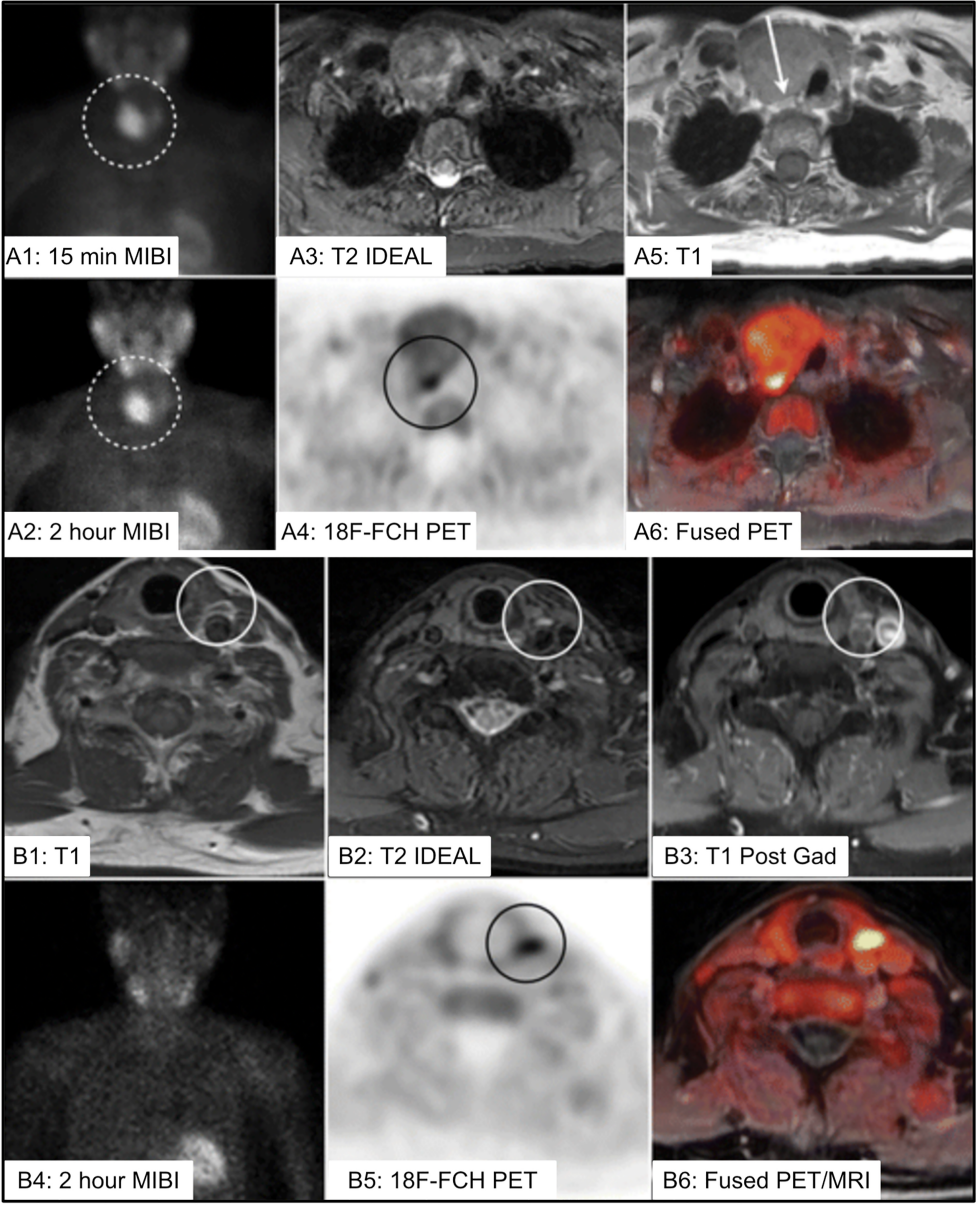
(A) 73 yo F with multinodular goiter and 5cm dominant nodule [(A1, A2), dotted circle] with uptake on 99mTc-sestamibi (MIBI) limiting ability to localize the PA. 18F-FCH PET/MR imaging identified PA posterior to the dominant nodule (A3–A6) [(A4), black circle, (A5), white arrow]. (B) 75 yo F with left PA identified on 4DMRI [(B1–B3), white circle], with inconclusive MIBI scan (B2), and correlative increased 18F-FCH uptake within the PA on PET/MRI imaging (B5, B6) [(B5), black circle]. IDEAL, Iterative decomposition of water and fat with echo asymmetry and least-squares estimation; 18F-FCH, 18F-fluorocholine; MIBI, 99mTc-sestamibi; PA, parathyroid adenoma. Reproduced with permission from: *Kluijfhout WP, Pasternak JD, Gosnell JE, Shen WT, Duh QY, Vriens MR, de Keizer B, Hope TA, Glastonbury CM, Pampaloni MH, Suh I. 18F Fluorocholine PET/MR Imaging in Patients with Primary Hyperparathyroidism and Inconclusive Conventional Imaging: A Prospective Pilot Study. Radiology. 2017 Aug;284(2):460-467. doi: 10.1148/radiol.2016160768. Epub 2017 Jan 25. PMID: 28121522*.
